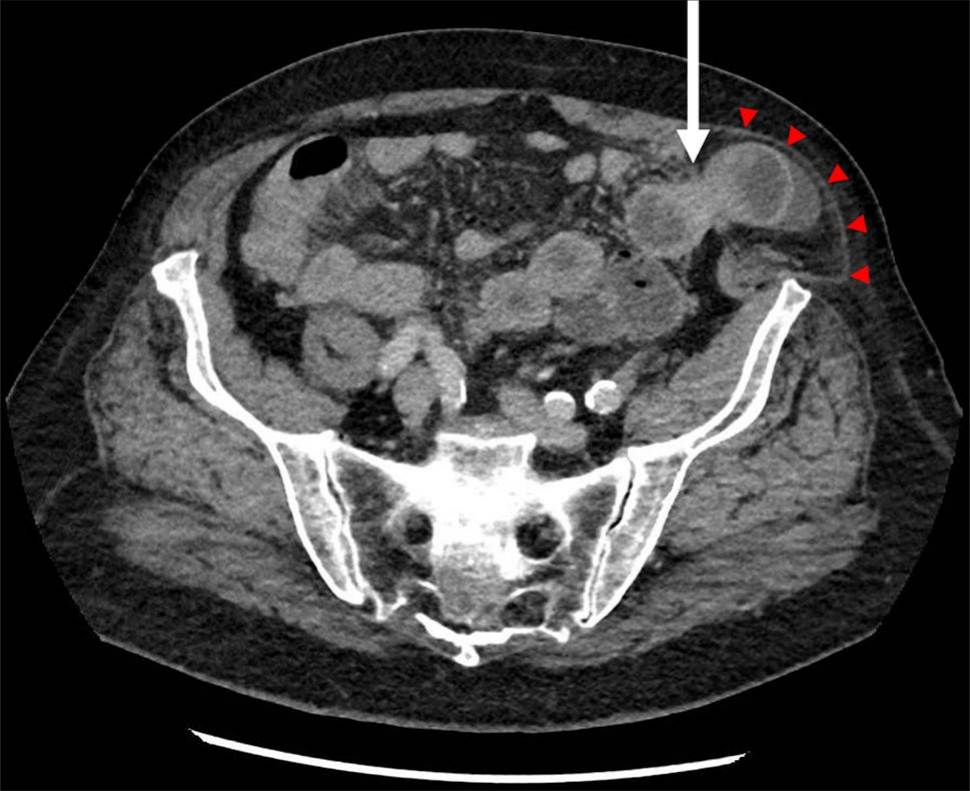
Spigelian Hernia on CT scan. Abdominal CT-scan depicting left-sided incarcerated Spigelian Hernia with small bowel content (arrow). Note the intact External oblique aponeurosis (arrowheads)
